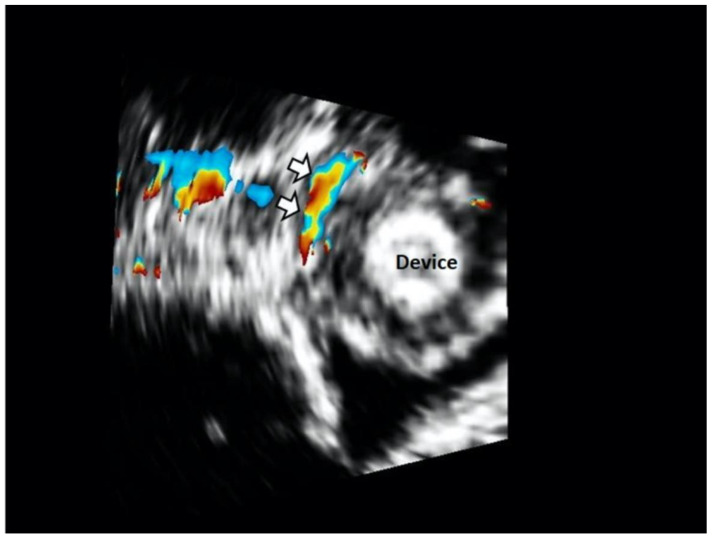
A 3D color Doppler en face view of the device of a patient with type C lesions demarcating the crescent-shaped nature of the leakage (white arrows).
